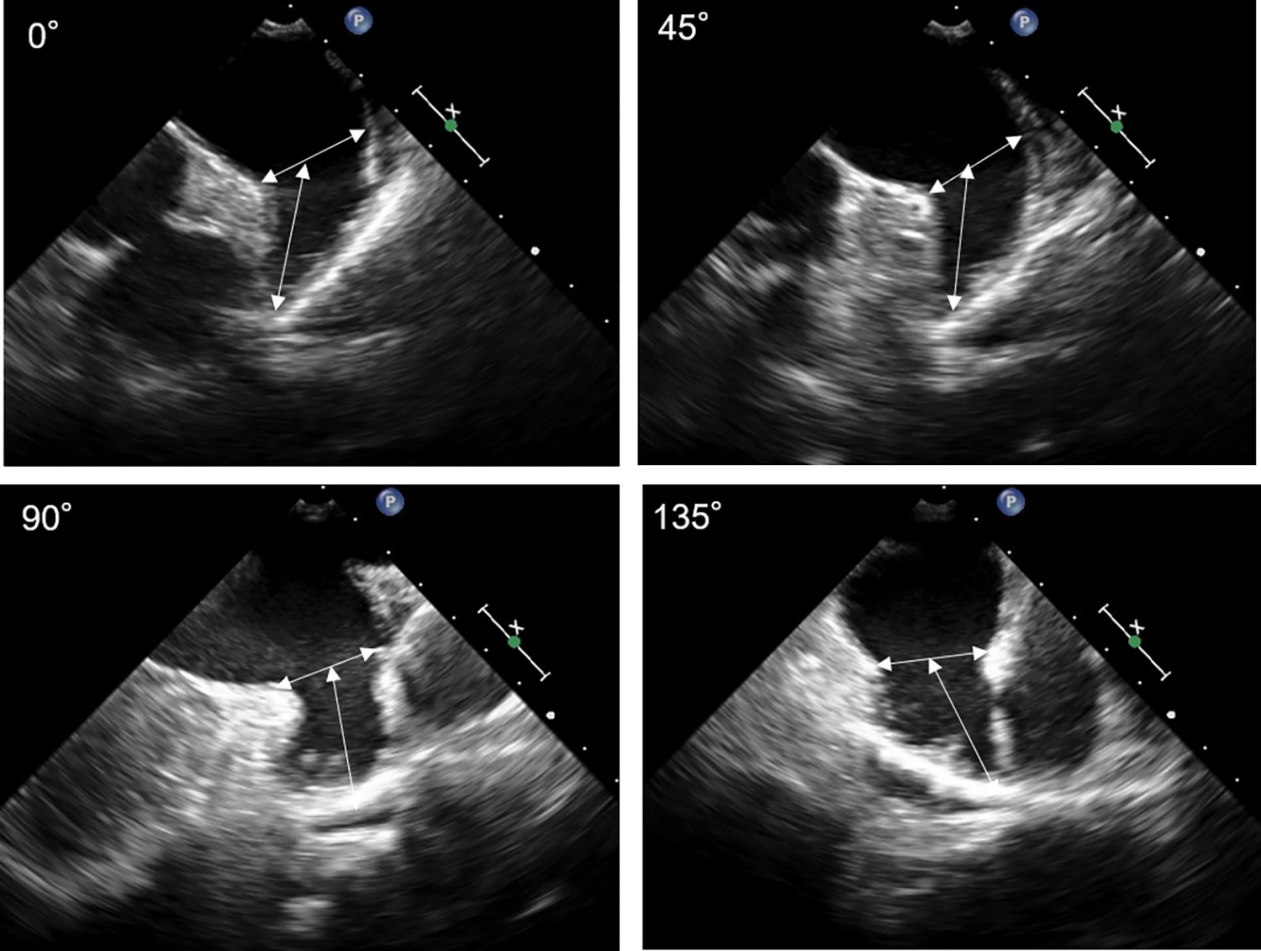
Transesophageal echocardiography measurement of left atrial appendage size. Left atrial appendage ostial diameter and depth were measured at 0°, 45°, 90°, and 135°.
